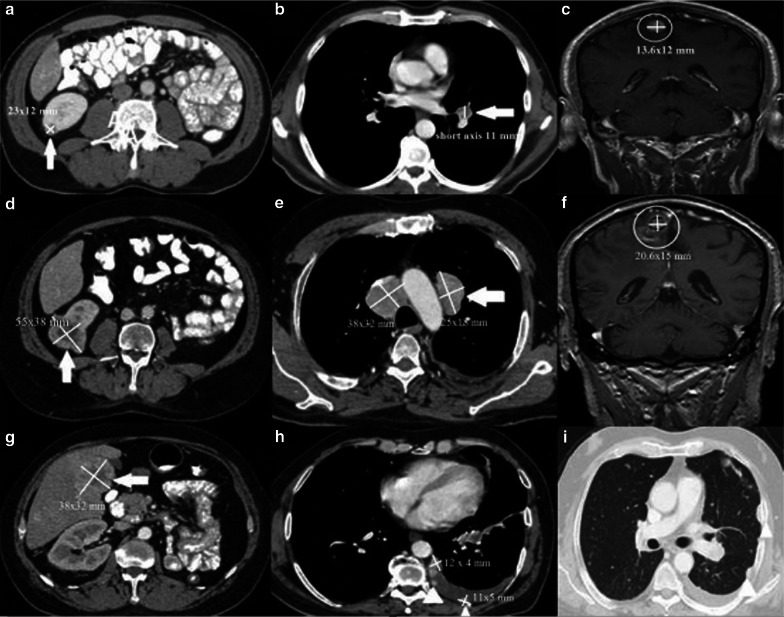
Continue from Fig. 6. a–c Neither CR nor PD was observed in the first control after initiation of chemotherapy treatment (arrows and circle). Immunotherapy treatment was started after this control imaging findings. d–f > 20% increase in the nadir of the sum of target lesions was observed (arrows and circle) g-i At the same control, new lesions were developed. Axial CT image shows a metastatic lesion in the right lobe of the liver (arrow) and multiple metastatic nodules in the left parietal pleura (arrowheads)
